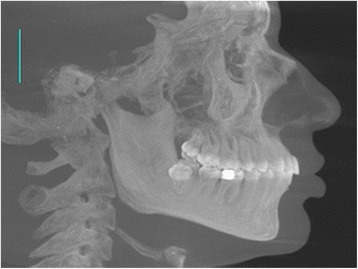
CBCT-synthesized lateral cephalometric radiograph
